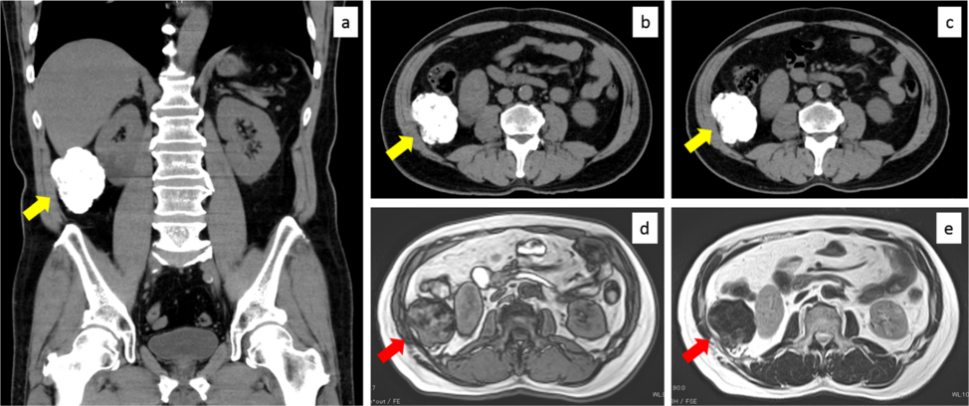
Preoperative images. a, b Images of plain computed tomography on the patient’s first visit. A mass with extremely high densities was located next to the undersurface of the right hepatic lobe and the right kidney (*yellow arrows*). It was 6.5 cm in the longest diameter. c An image of plain computed tomography 2 years after the patient’s first visit (*yellow arrow*). There were no remarkable changes of the mass in its appearance or size. d, e Magnetic resonance imaging on the patient’s first visit. The mass showed low signal intensities on both d T1- and e T2-weighted images (*red arrows*)
